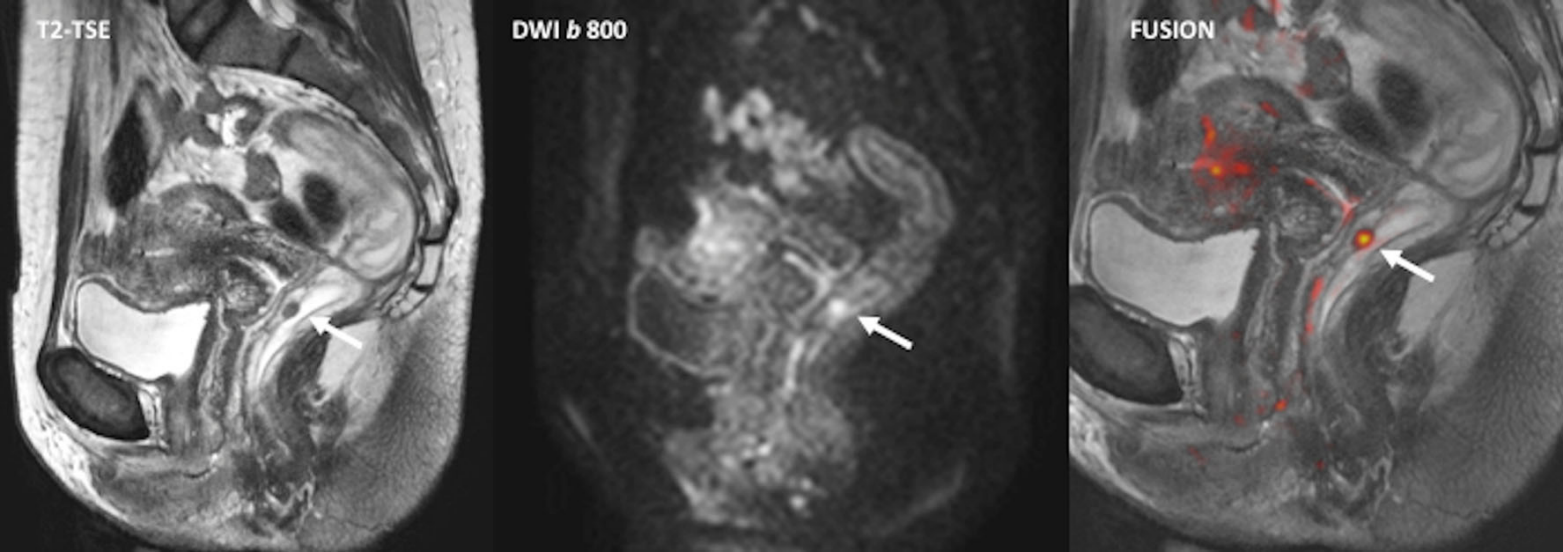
Diffusion for detecting colorectal tumours. A carcinoid tumour (white arrows) in a 44-year-old patient. Sagittal fast spin-echo T2-weighted image (left) and sagittal diffusion-weighted image with high b value (b = 800 s/mm^2^) (center) showed a rectal tumour nodule. Fused image (right) superimposing sagittal T2-weighted MR image and color-coded map derived from high-b-value diffusion-weighted image clearly delineated the tumour
